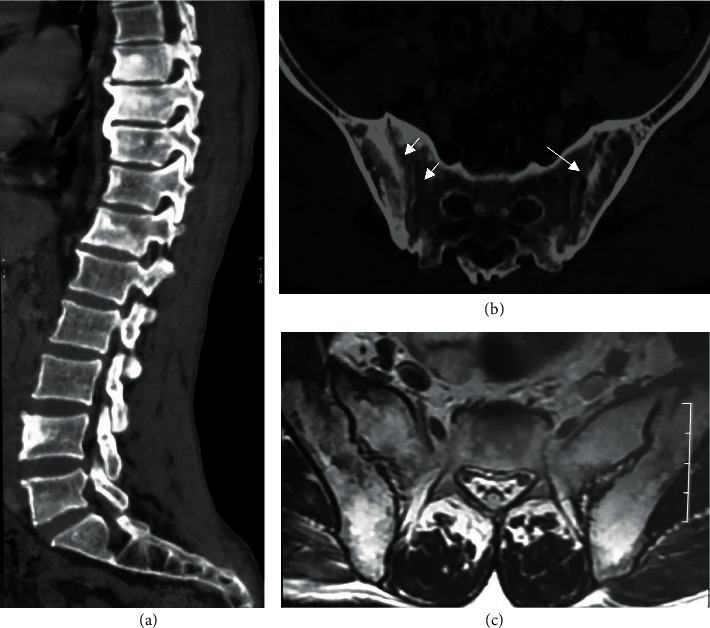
(a) Sagittal CT image showing osteosclerosis of vertebral bodies of D8 to D12 and L4 with paravertebral ossifications. (b) Axial CT image showing intra-articular bridges and multiple well-defined erosions with sclerotic margins. Bone sclerosis is blurred within the trabecular bone. (c) Magnetic resonance imaging axial T1 WI showing bilateral sacroiliitis with a fatty marrow signal.
